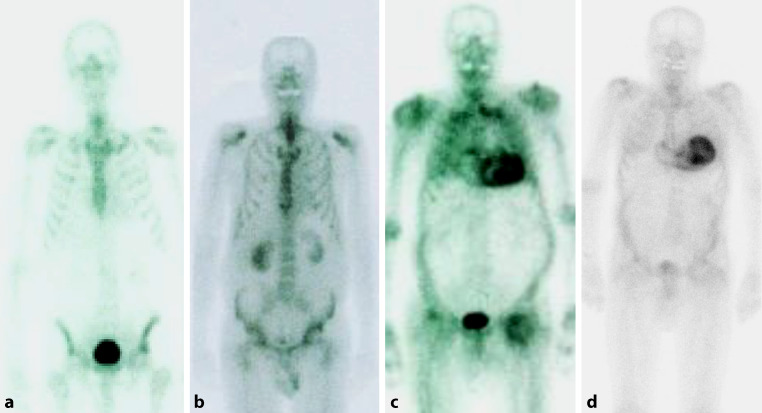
Whole-body scintigraphy using ^99m^Tc-labeled hydroxymethyl diphosphonate. a Healthy individual without any cardiac tracer uptake (Perugini grade 0). b Patient with cardiac light-chain amyloidosis (AL amyloidosis) and minor cardiac uptake (Perugini grade 1). c Patient with cardiac transthyretin amyloidosis (ATTR amyloidosis) and pronounced tracer uptake in the myocardium as well as attenuated osseous uptake (Perugini grade 2). d Patient with pronounced tracer uptake in the myocardium but only minor osseous uptake (Perugini grade 3)
